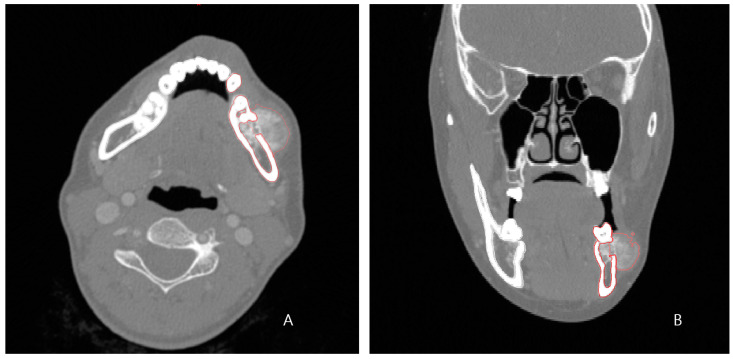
Preoperative enhanced CT (1.0 mm slice) scan showing the malignant tumor eroded the left buccal cortical bone and bulged. (A) axial view; (B) frontal view (red lines outline the lesion and surrounding tissue).
